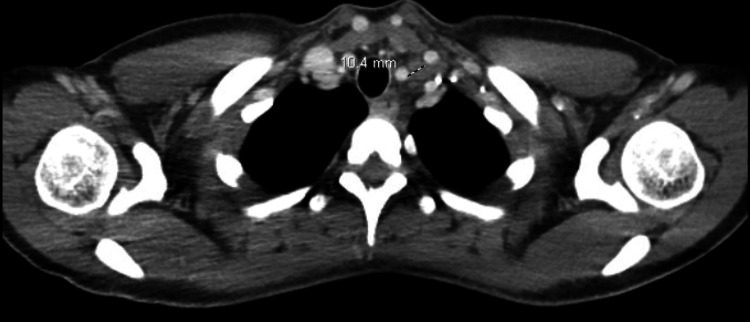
CT of the chest showing a mildly enlarged superior mediastinal lymph node between the left subclavian and left common carotid artery.
